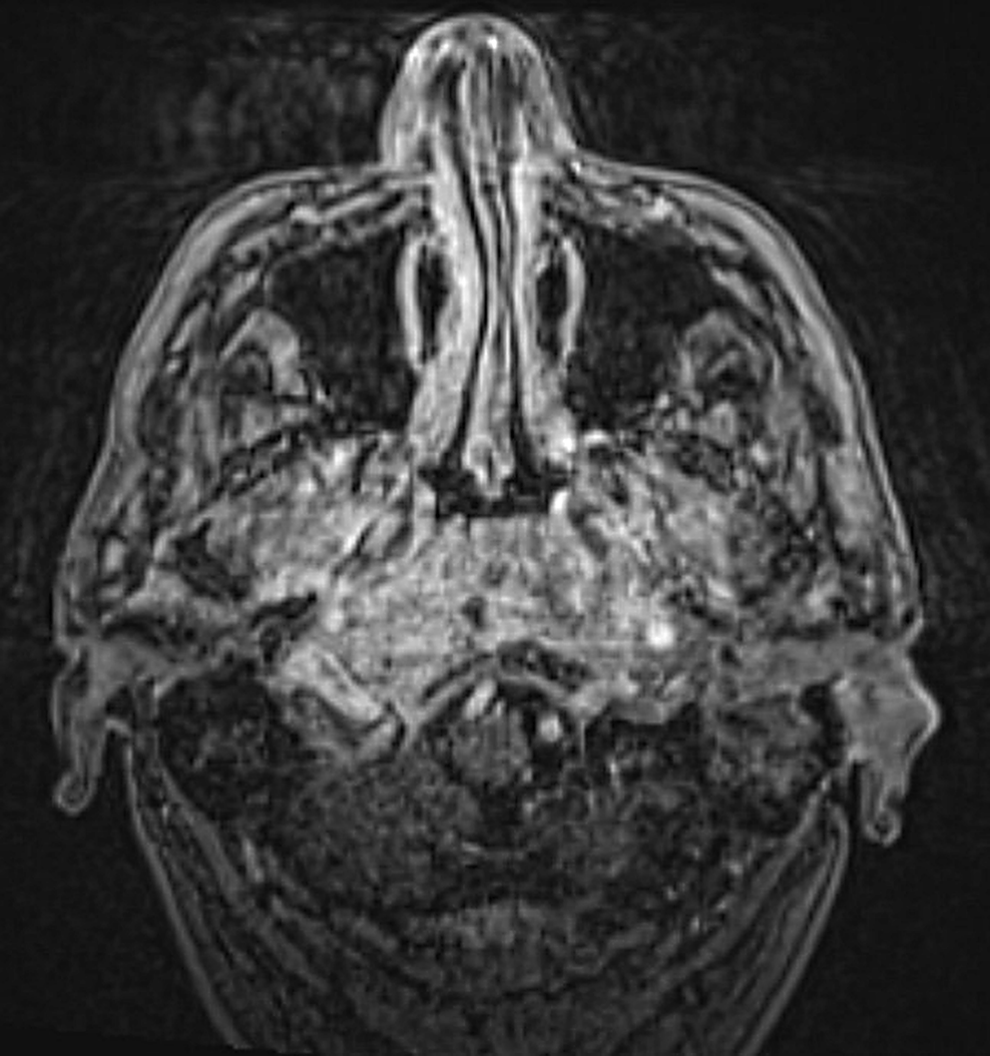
MRI examination at the start of treatment (ASBO)
